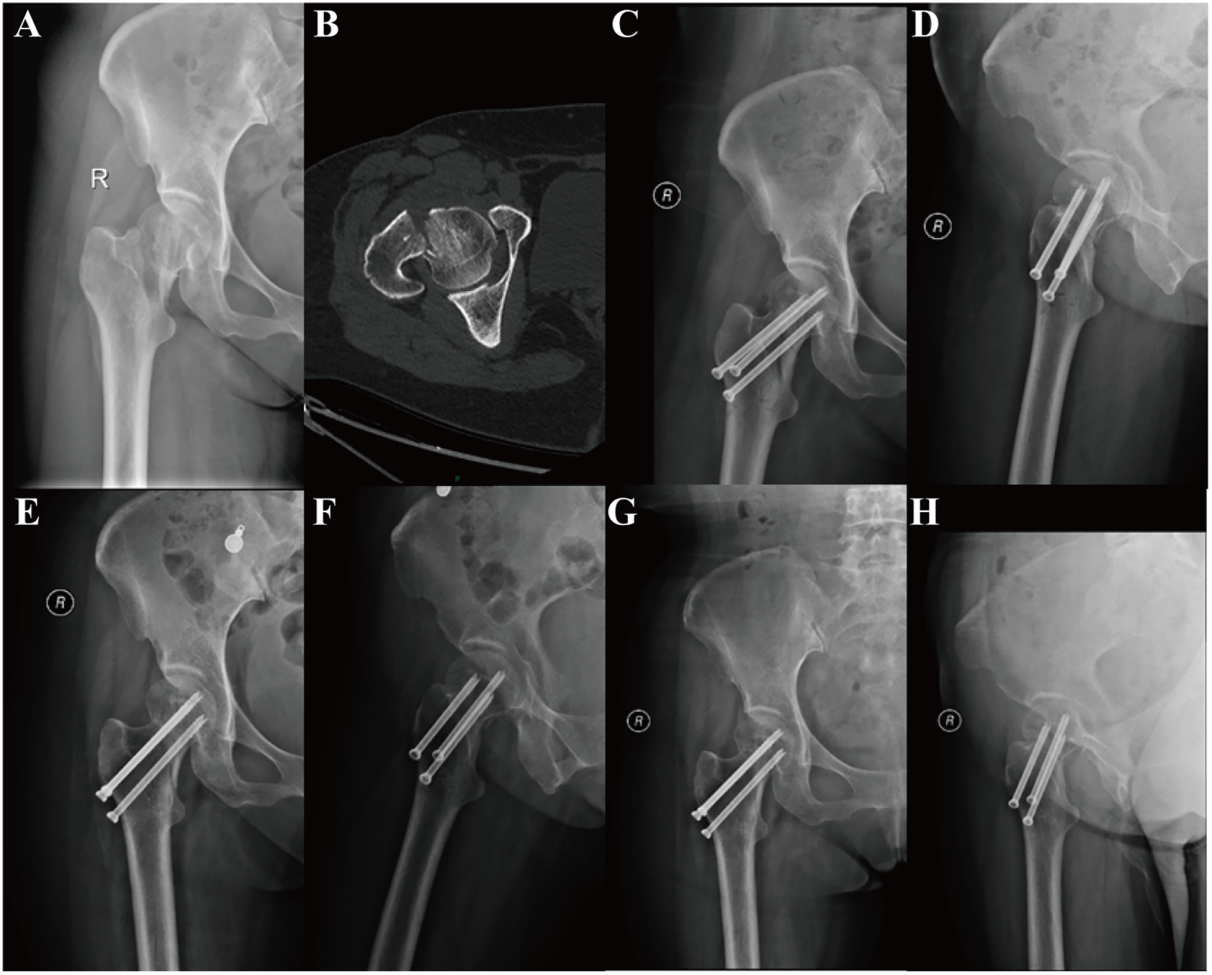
A 50-year-old female patient was admitted to the hospital for treatment of right hip pain and limited range of motion following a fall and underwent CCS treatment. (A,B) Pre-operative CT images of the patient showed a right femoral neck fracture, Garden type IV. (C,D) Review radiographs 1 day post-operatively in the anteroposterior and lateral positions showed good reduction. (E,F) Review radiographs 4 months post-operatively. (G,H) Review radiographs 13 months post-operatively.
